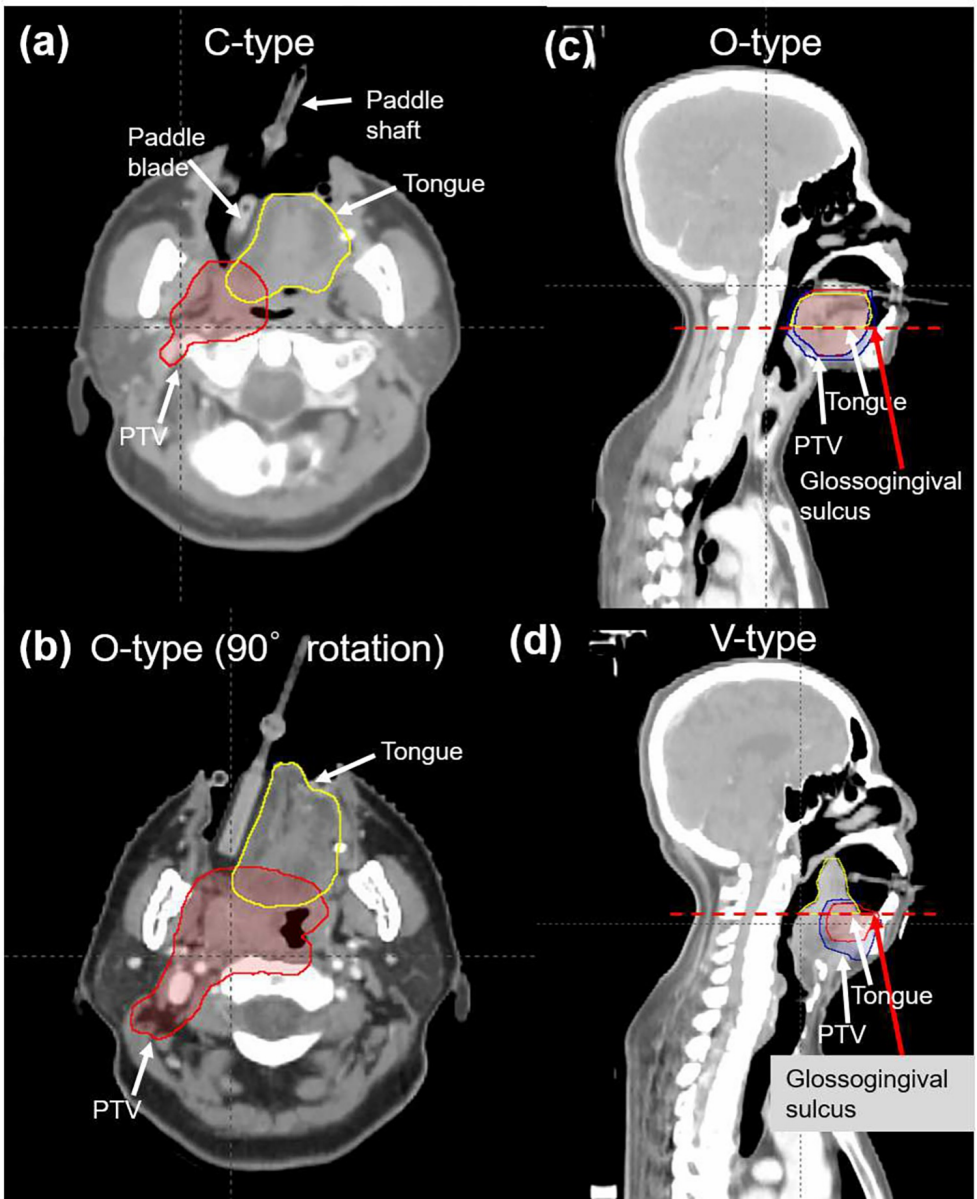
Planning CT images of patients with tongue-positioning devices. (a) axial view of C-type insertion, (b) axial view of O-type with 90° rotation insertion, (c) sagittal view of O-type insertion, and (d) sagittal view of V-type insertion. The red dotted horizontal line shows the bottom plane of the tongue.
